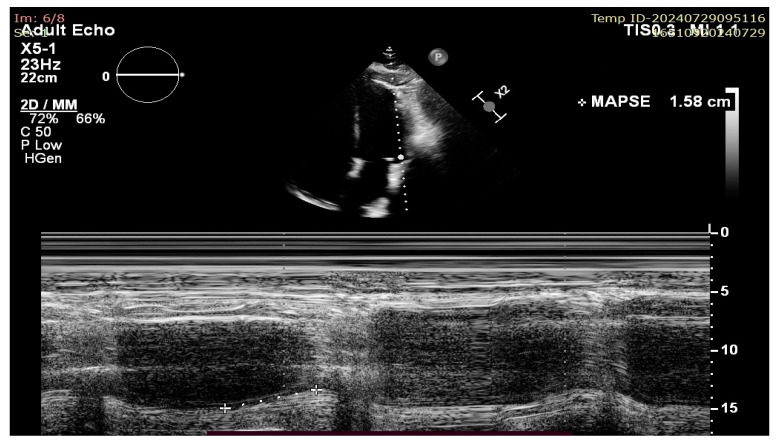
Lateral MAPSE measurement in the apical four chamber view.
